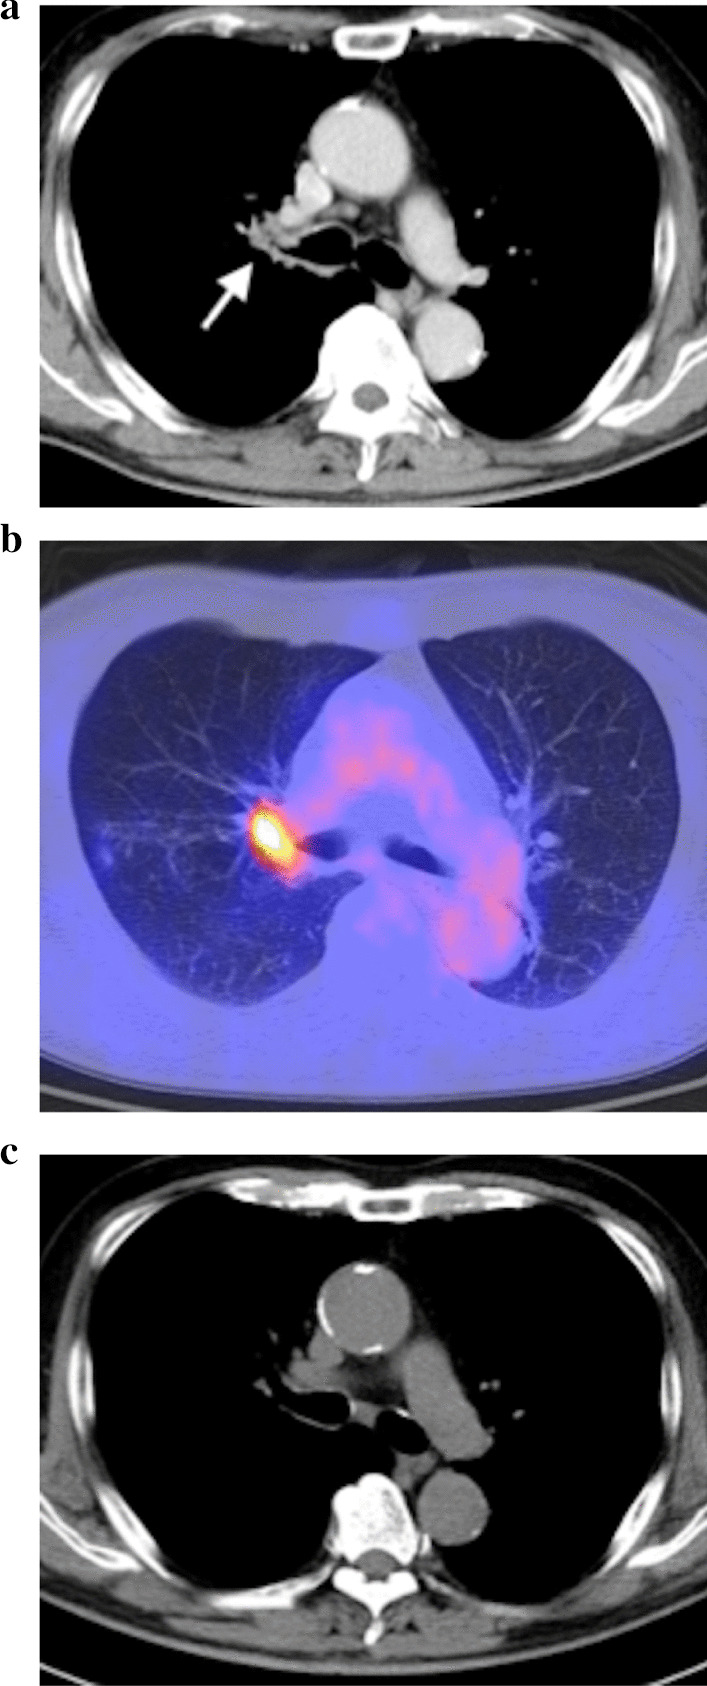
a Enhanced computed tomography showing a contrast effect in the tumor in the right pulmonary hilum and stenosis of the right main bronchus (arrow). b Positron emission tomography-computed tomography showing a tumor with a standardized uptake value of 8.5 in the pulmonary hilum. c Preoperative computed tomography showing semicircular calcification of the ascending aorta
